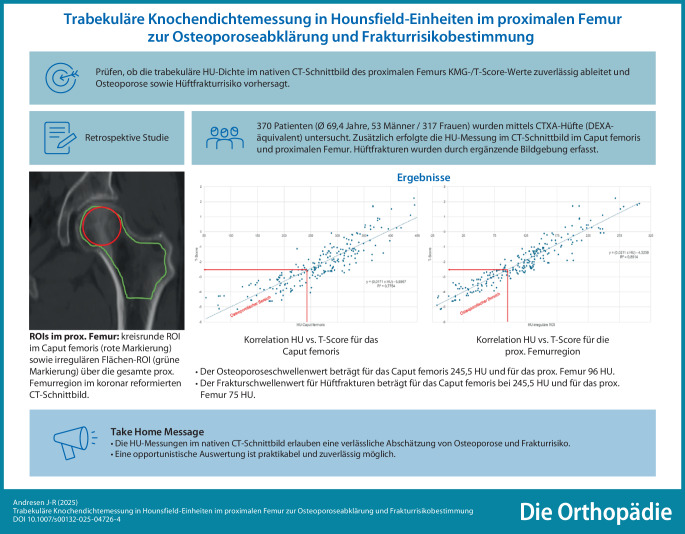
Am häufigsten kommt es zu Frakturen der Hüfte bei älteren Patienten nach einem Bagatelltrauma. Meist ist ein erhöhtes Frakturrisiko aufgrund einer vorhandenen Osteoporose nicht bekannt und somit liegt auch keine antiosteoporotische medikamentöse Therapie vor. Aus unterschiedlichen medizinischen Indikationen liegen häufig CT-Untersuchungen der Beckenregion vor, hier könnte die Bestimmung der trabekulären Knochendichte in Hounsfield-Einheiten (HU) im proximalen Femur eine Abschätzung einer vorliegenden Osteopenie/Osteoporose ohne zusätzliche Strahlenbelastung ermöglichen.
